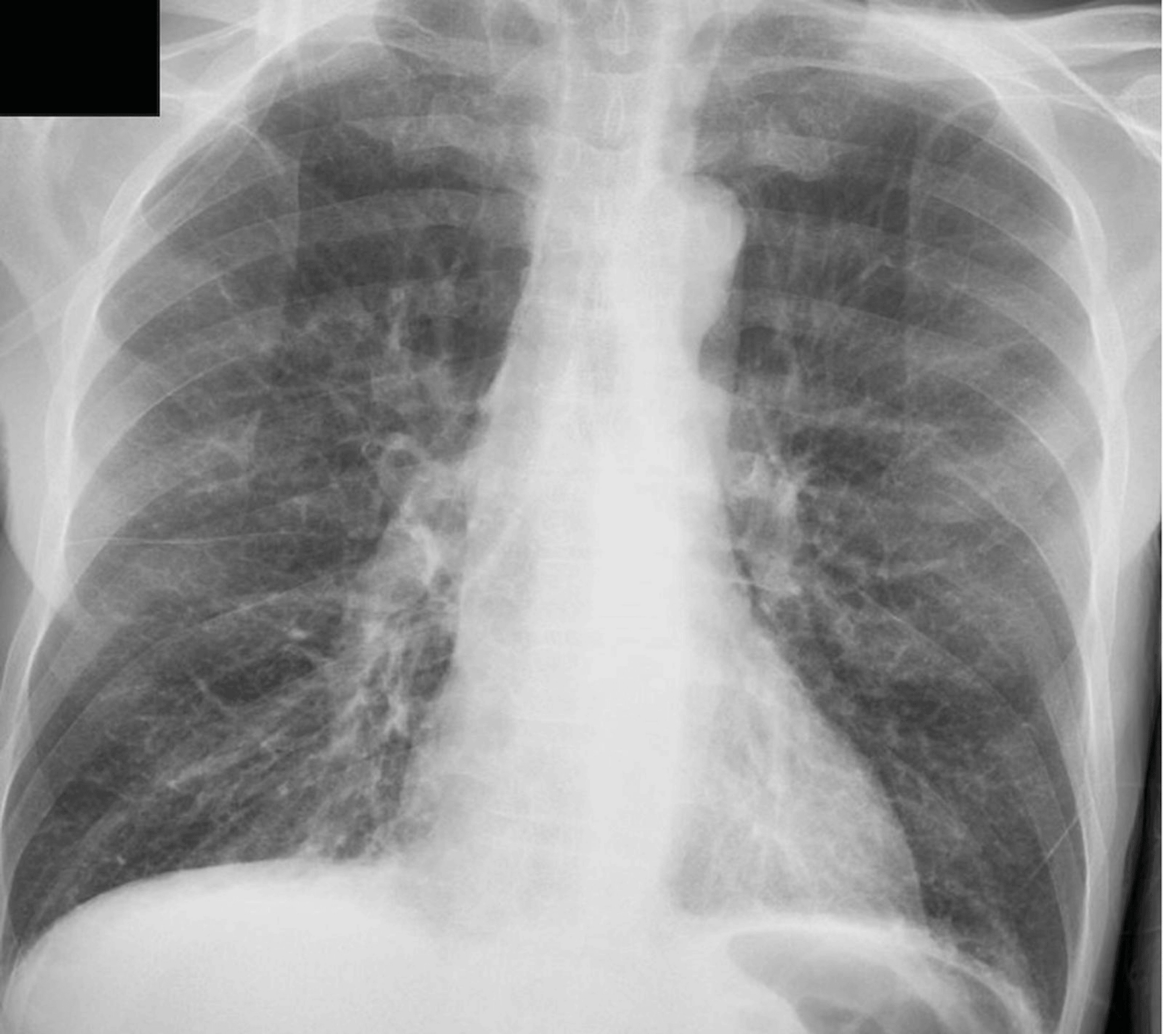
Chest X-ray showing pulmonary hyperinflation and pulmonary emphysema.
